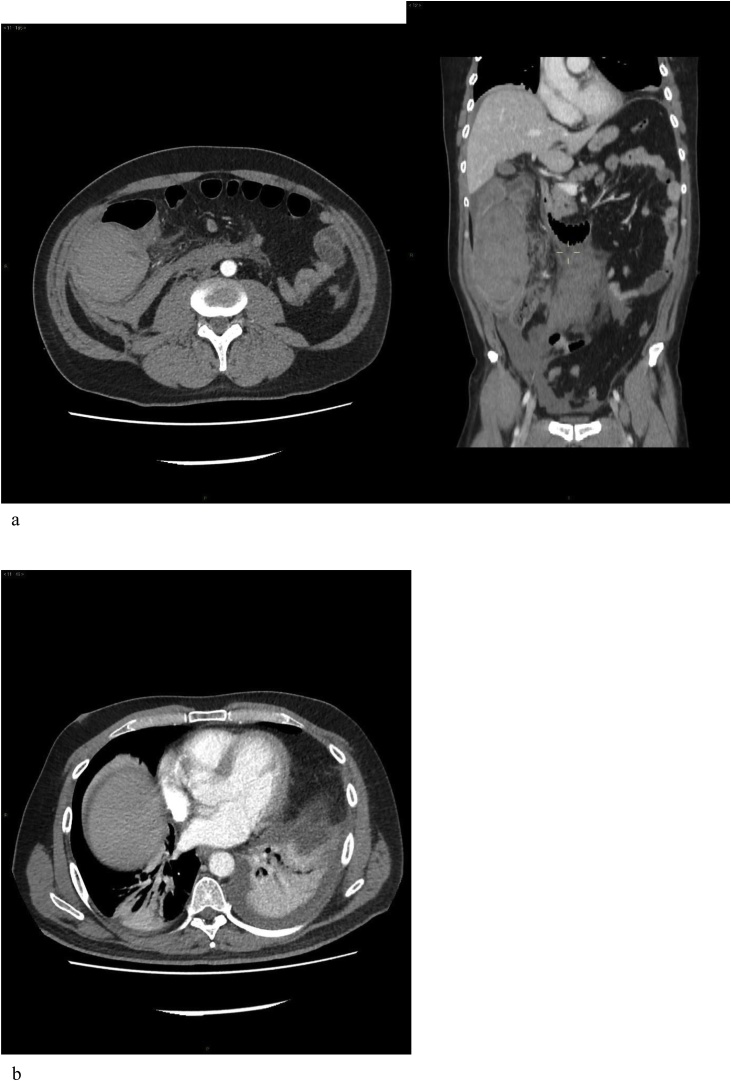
(a) CT scan revealing marked thickening of right colon. (b) Pleural effusion with pulmonary atelectasia.
